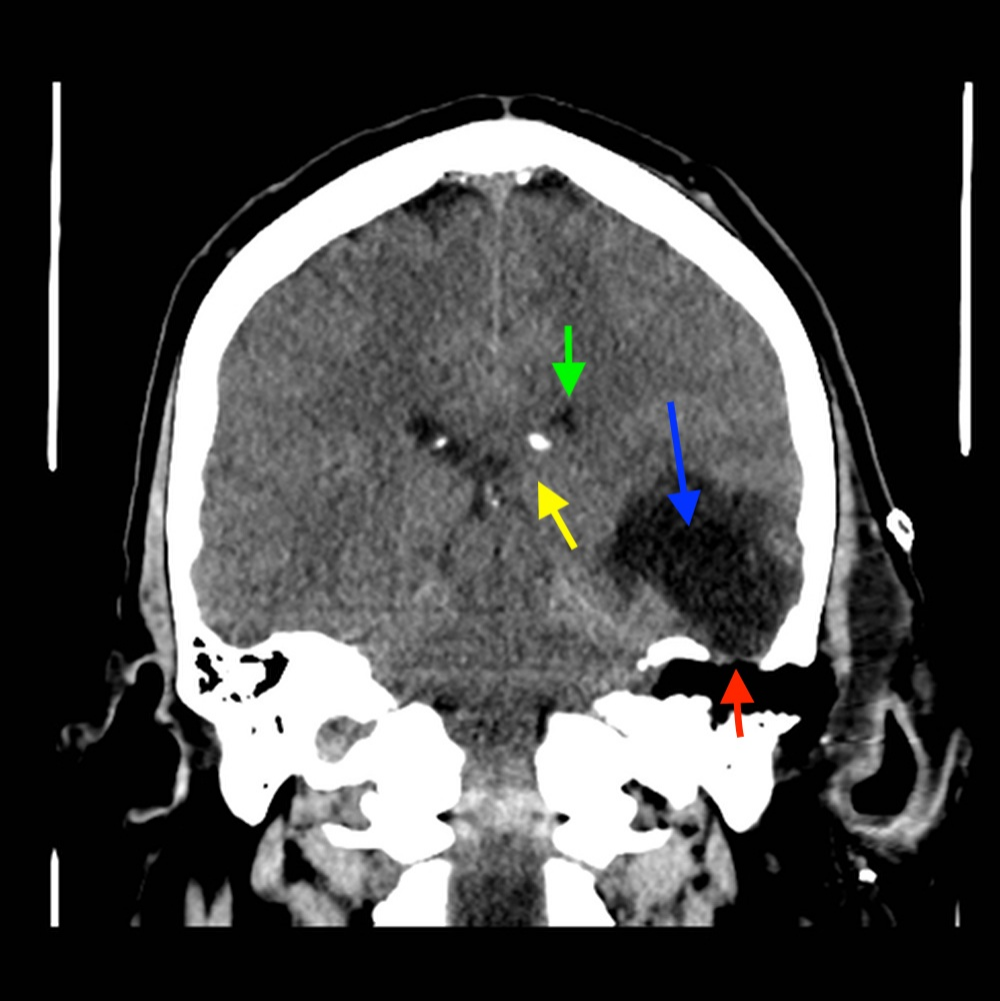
Coronal unenhanced CT of the head at the level of EAM: large lucency left temporal lobe (blue arrow), tegmen tympani defect (red arrow), mass effect on left lateral ventricle (green arrow) and on medial temporal lobe (yellow arrow). CT - computed tomography, EAM - external acoustic meatus
